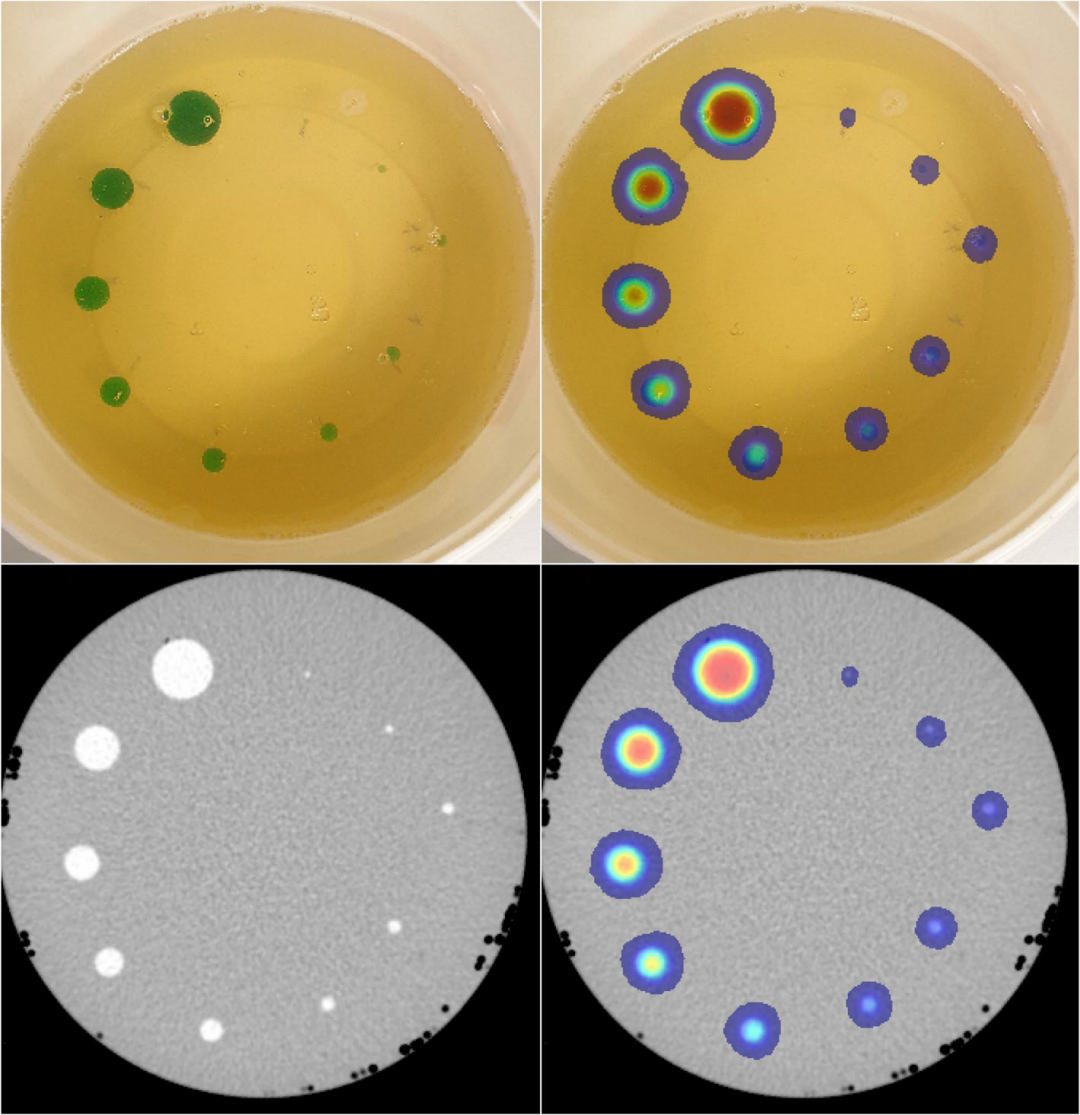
Spheres in gelatin mixture (a), spheres in gelatin mixture with PET signal overlaid (b), CT of spheres (c) and PET/CT images of spheres (d)
